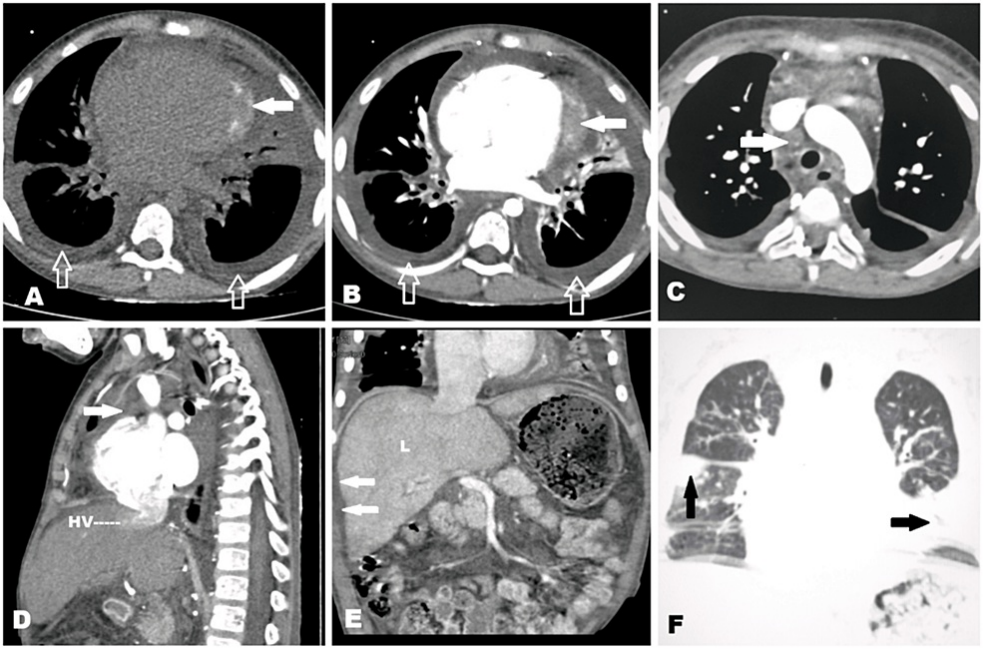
(A) Plain CT of the chest in the axial section showing a thickened pericardium with calcifications (solid white arrow) and bilateral pleural effusion (open arrow). (B) Contrast-enhanced CT in the axial section showing non-enhancing pericardial thickening (solid arrow) with bilateral pleural effusion (open arrow). (C) Contrast-enhanced CT in the axial section showing an enlarged mediastinal node (solid white arrow). (D) Contrast-enhanced CT in the sagittal section showing reflux of the contrast into the hepatic vein (HV) with enlarged mediastinal node (solid white arrow). (E) Coronal reconstructed contrast-enhanced CT of the abdomen showing minimal ascites (solid white arrow) with hepatomegaly (L). (F) Coronal reconstructed CT of the chest in lung window showing consolidation in both lung fields (black arrow).
